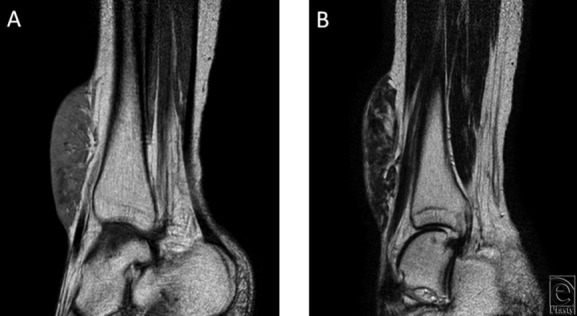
Magnetic resonance imaging findings. (a) T1 hypointensity and (b) T2 hyperintensity, irregular border on the deep side, and normal vessels and fat running through the mass.
